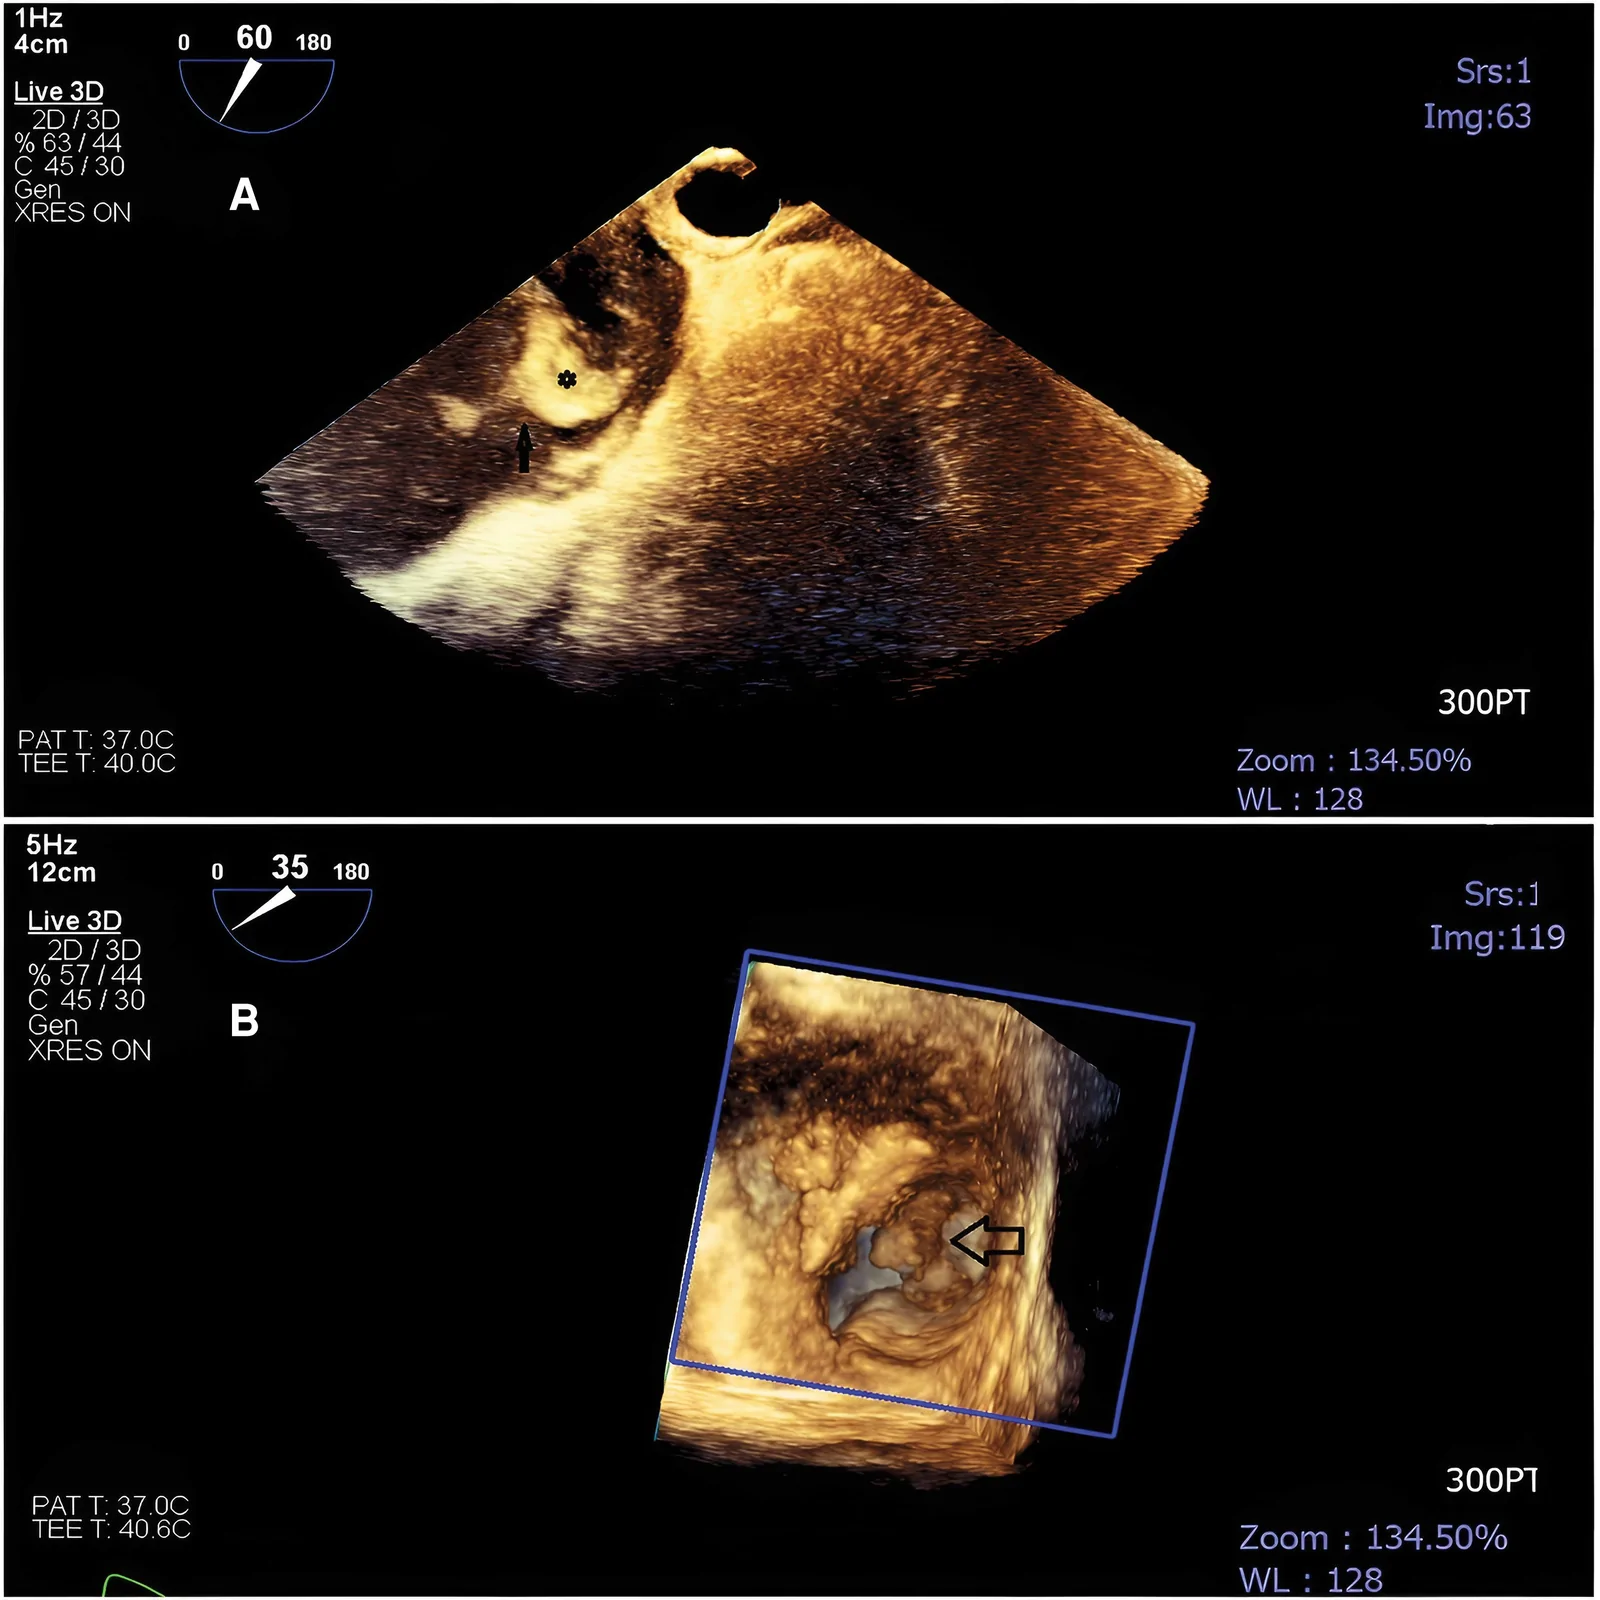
3D transoesophageal echocardiography (3D-TEE), (*A*) 60° en-face view and (*B*) 30° cropped view demonstrating a bicuspid pulmonary valve with multiple large, irregular, highly mobile vegetations (arrows) causing marked anatomical distortion.
